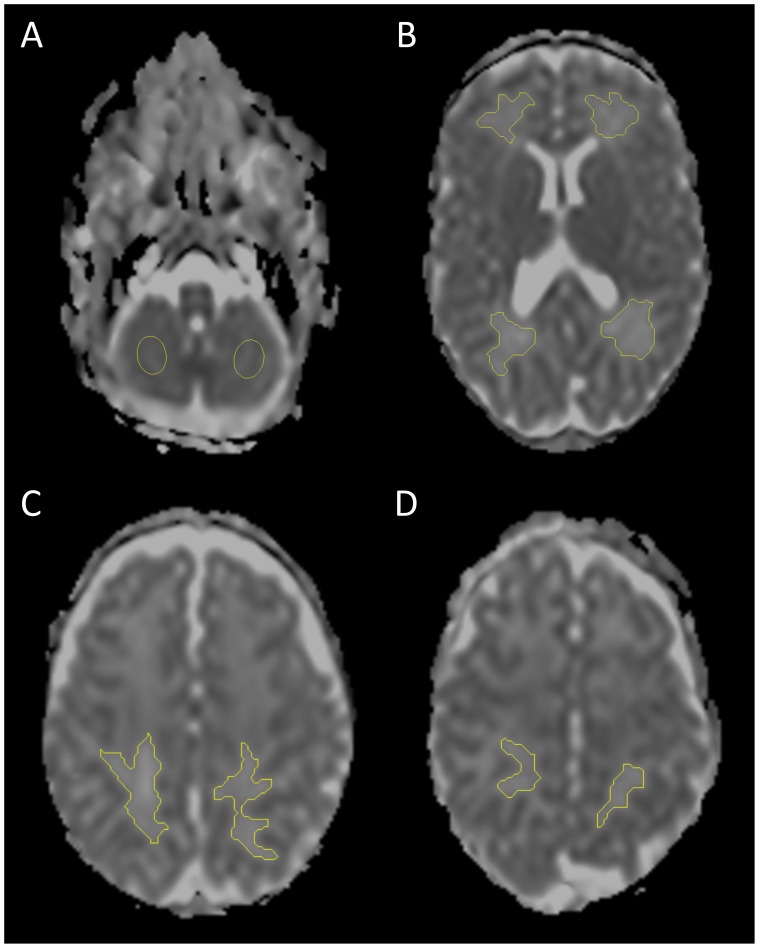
Evaluation of ADC values on term DWI in ten ROIs in (A) cerebellum and (B) frontal, occipital, (C) parieto-occipital and (D) parietal WM.
